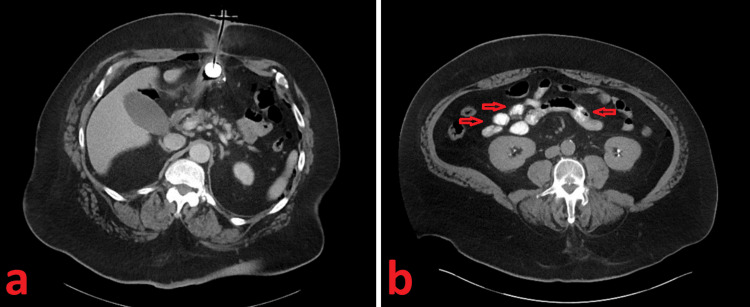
Computed tomography (CT) scan of the abdomen and pelvis with oral contrast Axial images of oral contrast-enhanced computed tomography of the abdomen and pelvis demonstrating (A) appropriate positioning of the PEG tube without evidence of extravasation of contrast and (B) contrast within loops of the small bowel, again without any evidence of extravasation.
